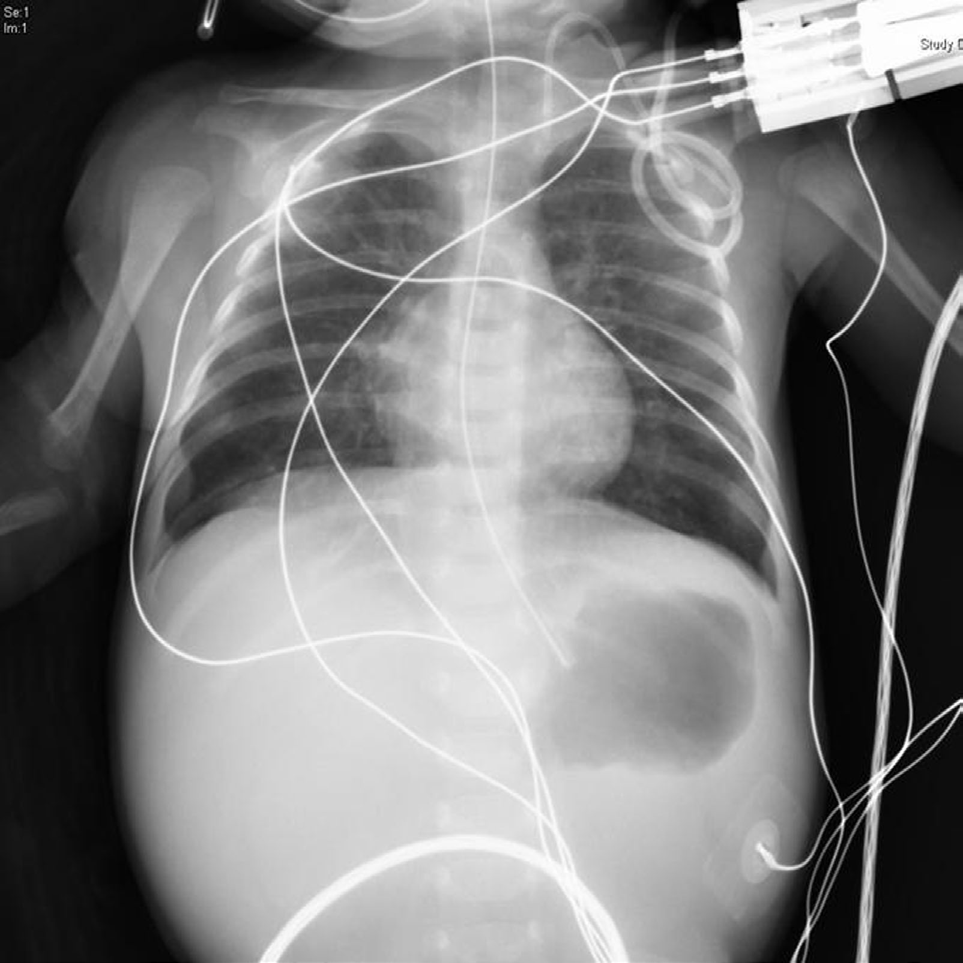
At 1 day of age, congenital pyloric atresia and narrow thymus shadow in her middle mediastinum were noted on a plain X-ray.
